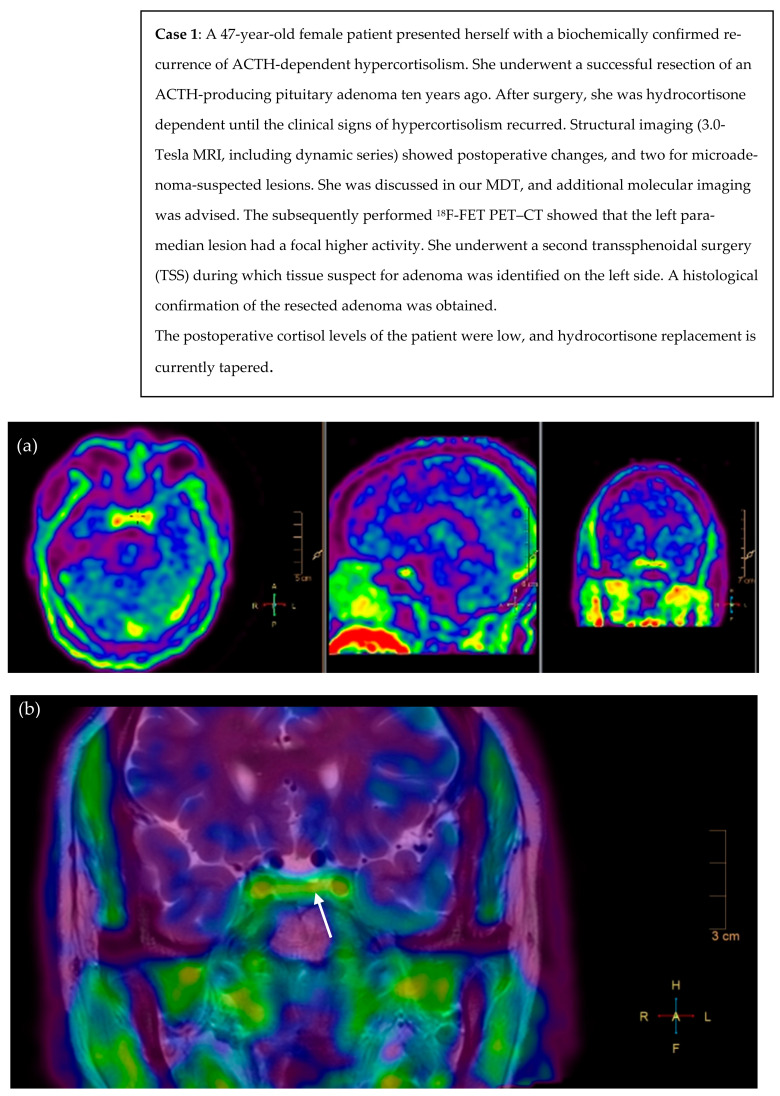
^18^F-FET PET scan and fusion of PET with T2 MR images reveal asymmetrical focal high uptake (in orange) in a pituitary adenoma left paramedian, as shown with the crosshair (a) and white arrow (b). (a) axial, sagittal, and coronal view of PET scan after infusion of 219 MBq ^18^F-FET, (b) fusion of PET/MRI (T2-weighted coronal view, pre-gadolinium). L = left, R = right, H = head, F = feet.
